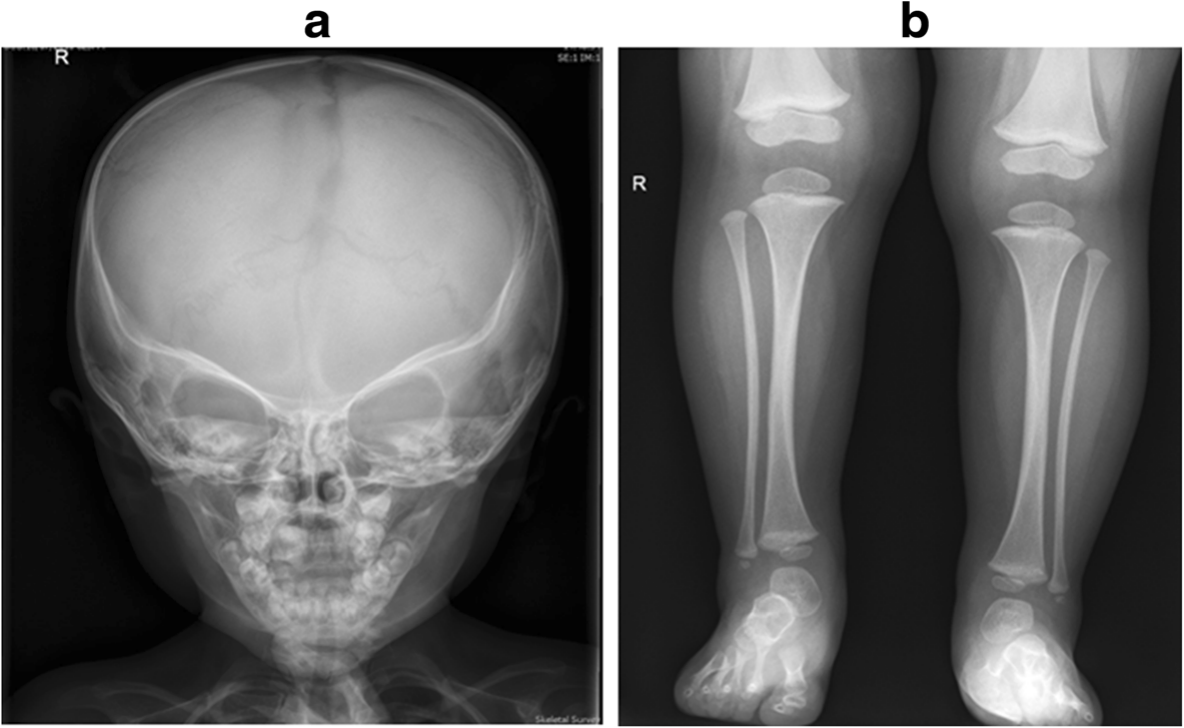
Figure 6a shows relatively poor ossification of skull vault, patent metopic suture, widely separated sagittal sutures on anteroposterior view of X-ray Skull. Figure 6b shows tubulated long bones with reduced medullary space and cortical thickening on anteroposterior view X-ray Tibia
